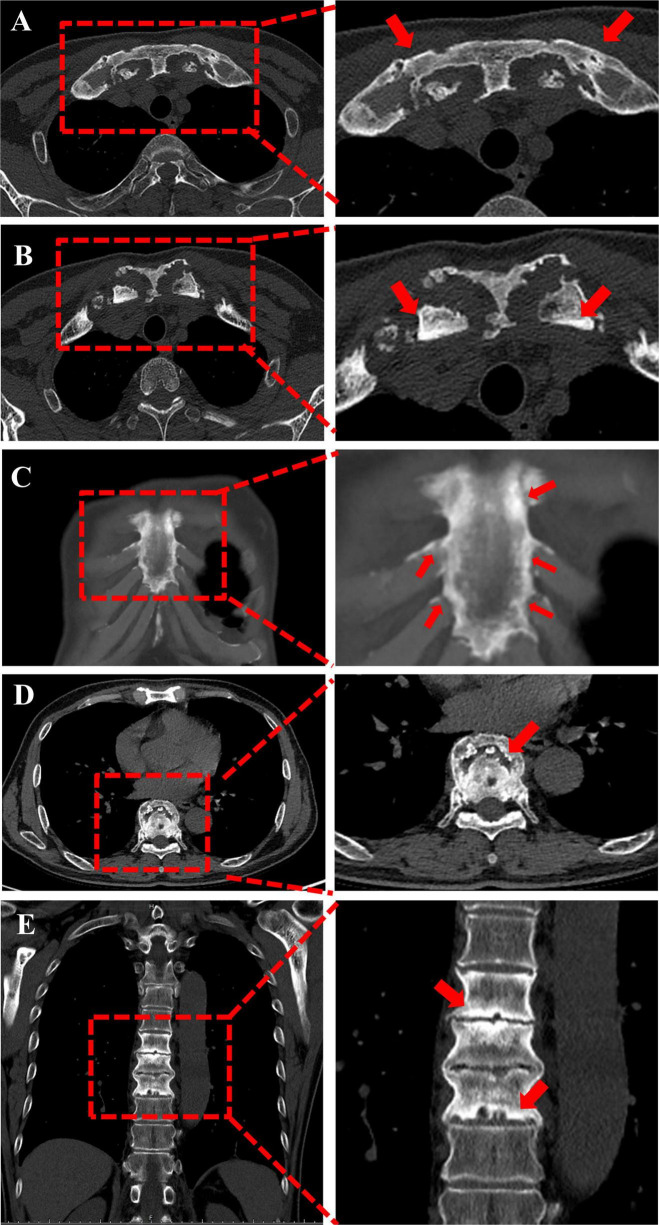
Imaging features of the sternum, clavicles and spine in the present case. CT images were obtained using a bone window setting (window level, C = 500, window width, *W* = 1,500). (A) Axial CT shows multiple irregular “worm-eaten” osteolytic lesions in the sternum and bilateral clavicles (arrows). (B) Sclerosis and hyperostosis of the sternum and bilateral clavicles are present (arrows). (C) Irregular margins of the sternoclavicular joints, sternocostal joints and vertebral endplates are seen. (D) Axial images show multiple osteolytic lesions in the thoracic vertebrae (arrows). (E) Coronal images show multiple irregular osteolytic lesions in the thoracic and lumbar vertebrae (arrows).
